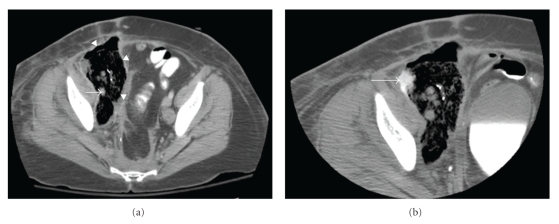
Contrast-enhanced pelvic CT (a) reveals 12 × 7 cm air- and fluid-filled abscess (arrowheads) in abdominal right lower quadrant. Encasement of right external iliac artery (arrow) by abscess noted. (b) Presence of bowel contrast (arrow) within collection confirms fistula to colon.
